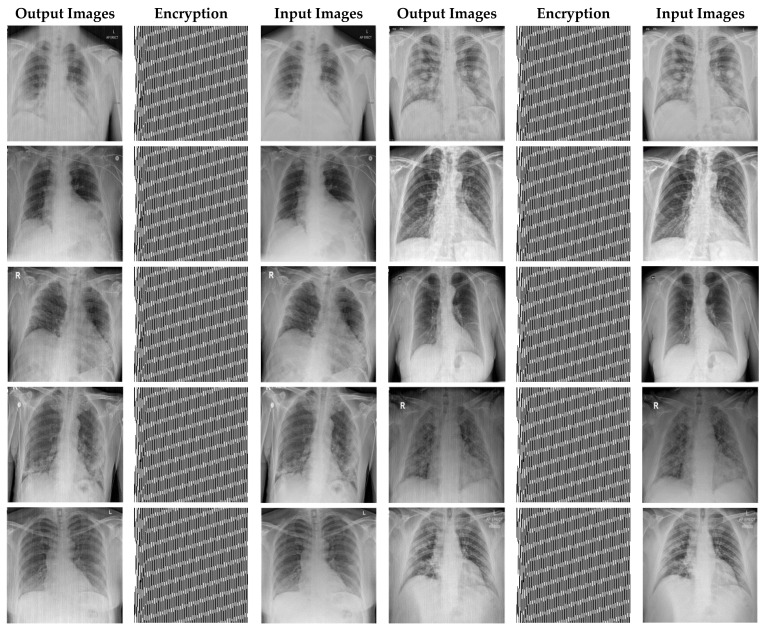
X-ray images encryption using the proposed method.
